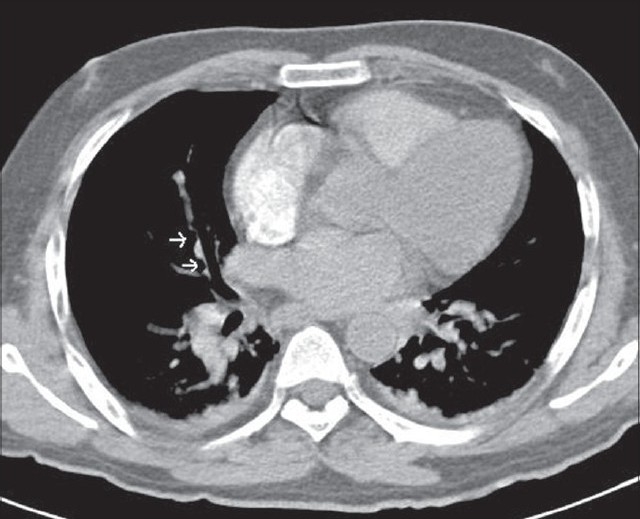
CT pulmonary angiography scan showing the filling defects (marked by arrows) in the right lower lobe segmental artery, which is suggestive of pulmonary embolism
